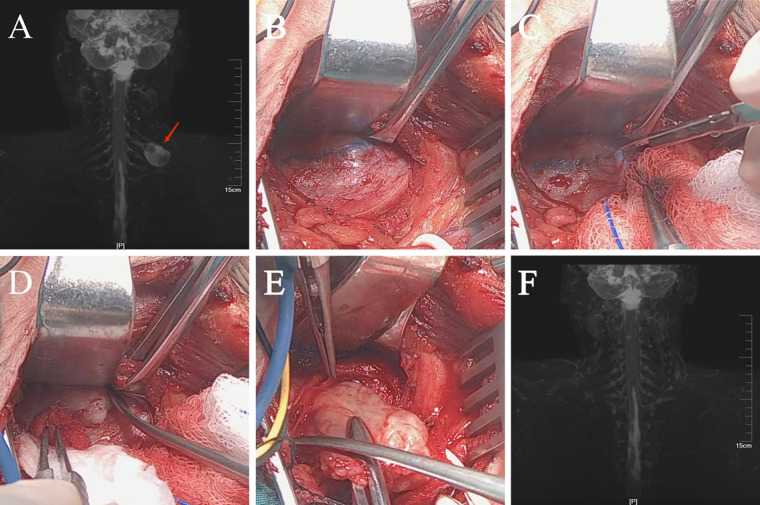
Brachial plexus schwannoma. (A) Magnetic resonance imaging (MRI) shows a round mass approximately 3.2 cm × 2.4 cm in size (arrow) in the left supraclavicular fossa. (B) The probe clearly identified the neural course and marked the incision route. (C–E) Nerve tracts were separated, and the tumor was excised intact from within the capsule. (F) One-year postoperative follow-up showed no abnormal signal in the surgical area. The morphology of and the signal from the brachial plexus showed no abnormality as well.
